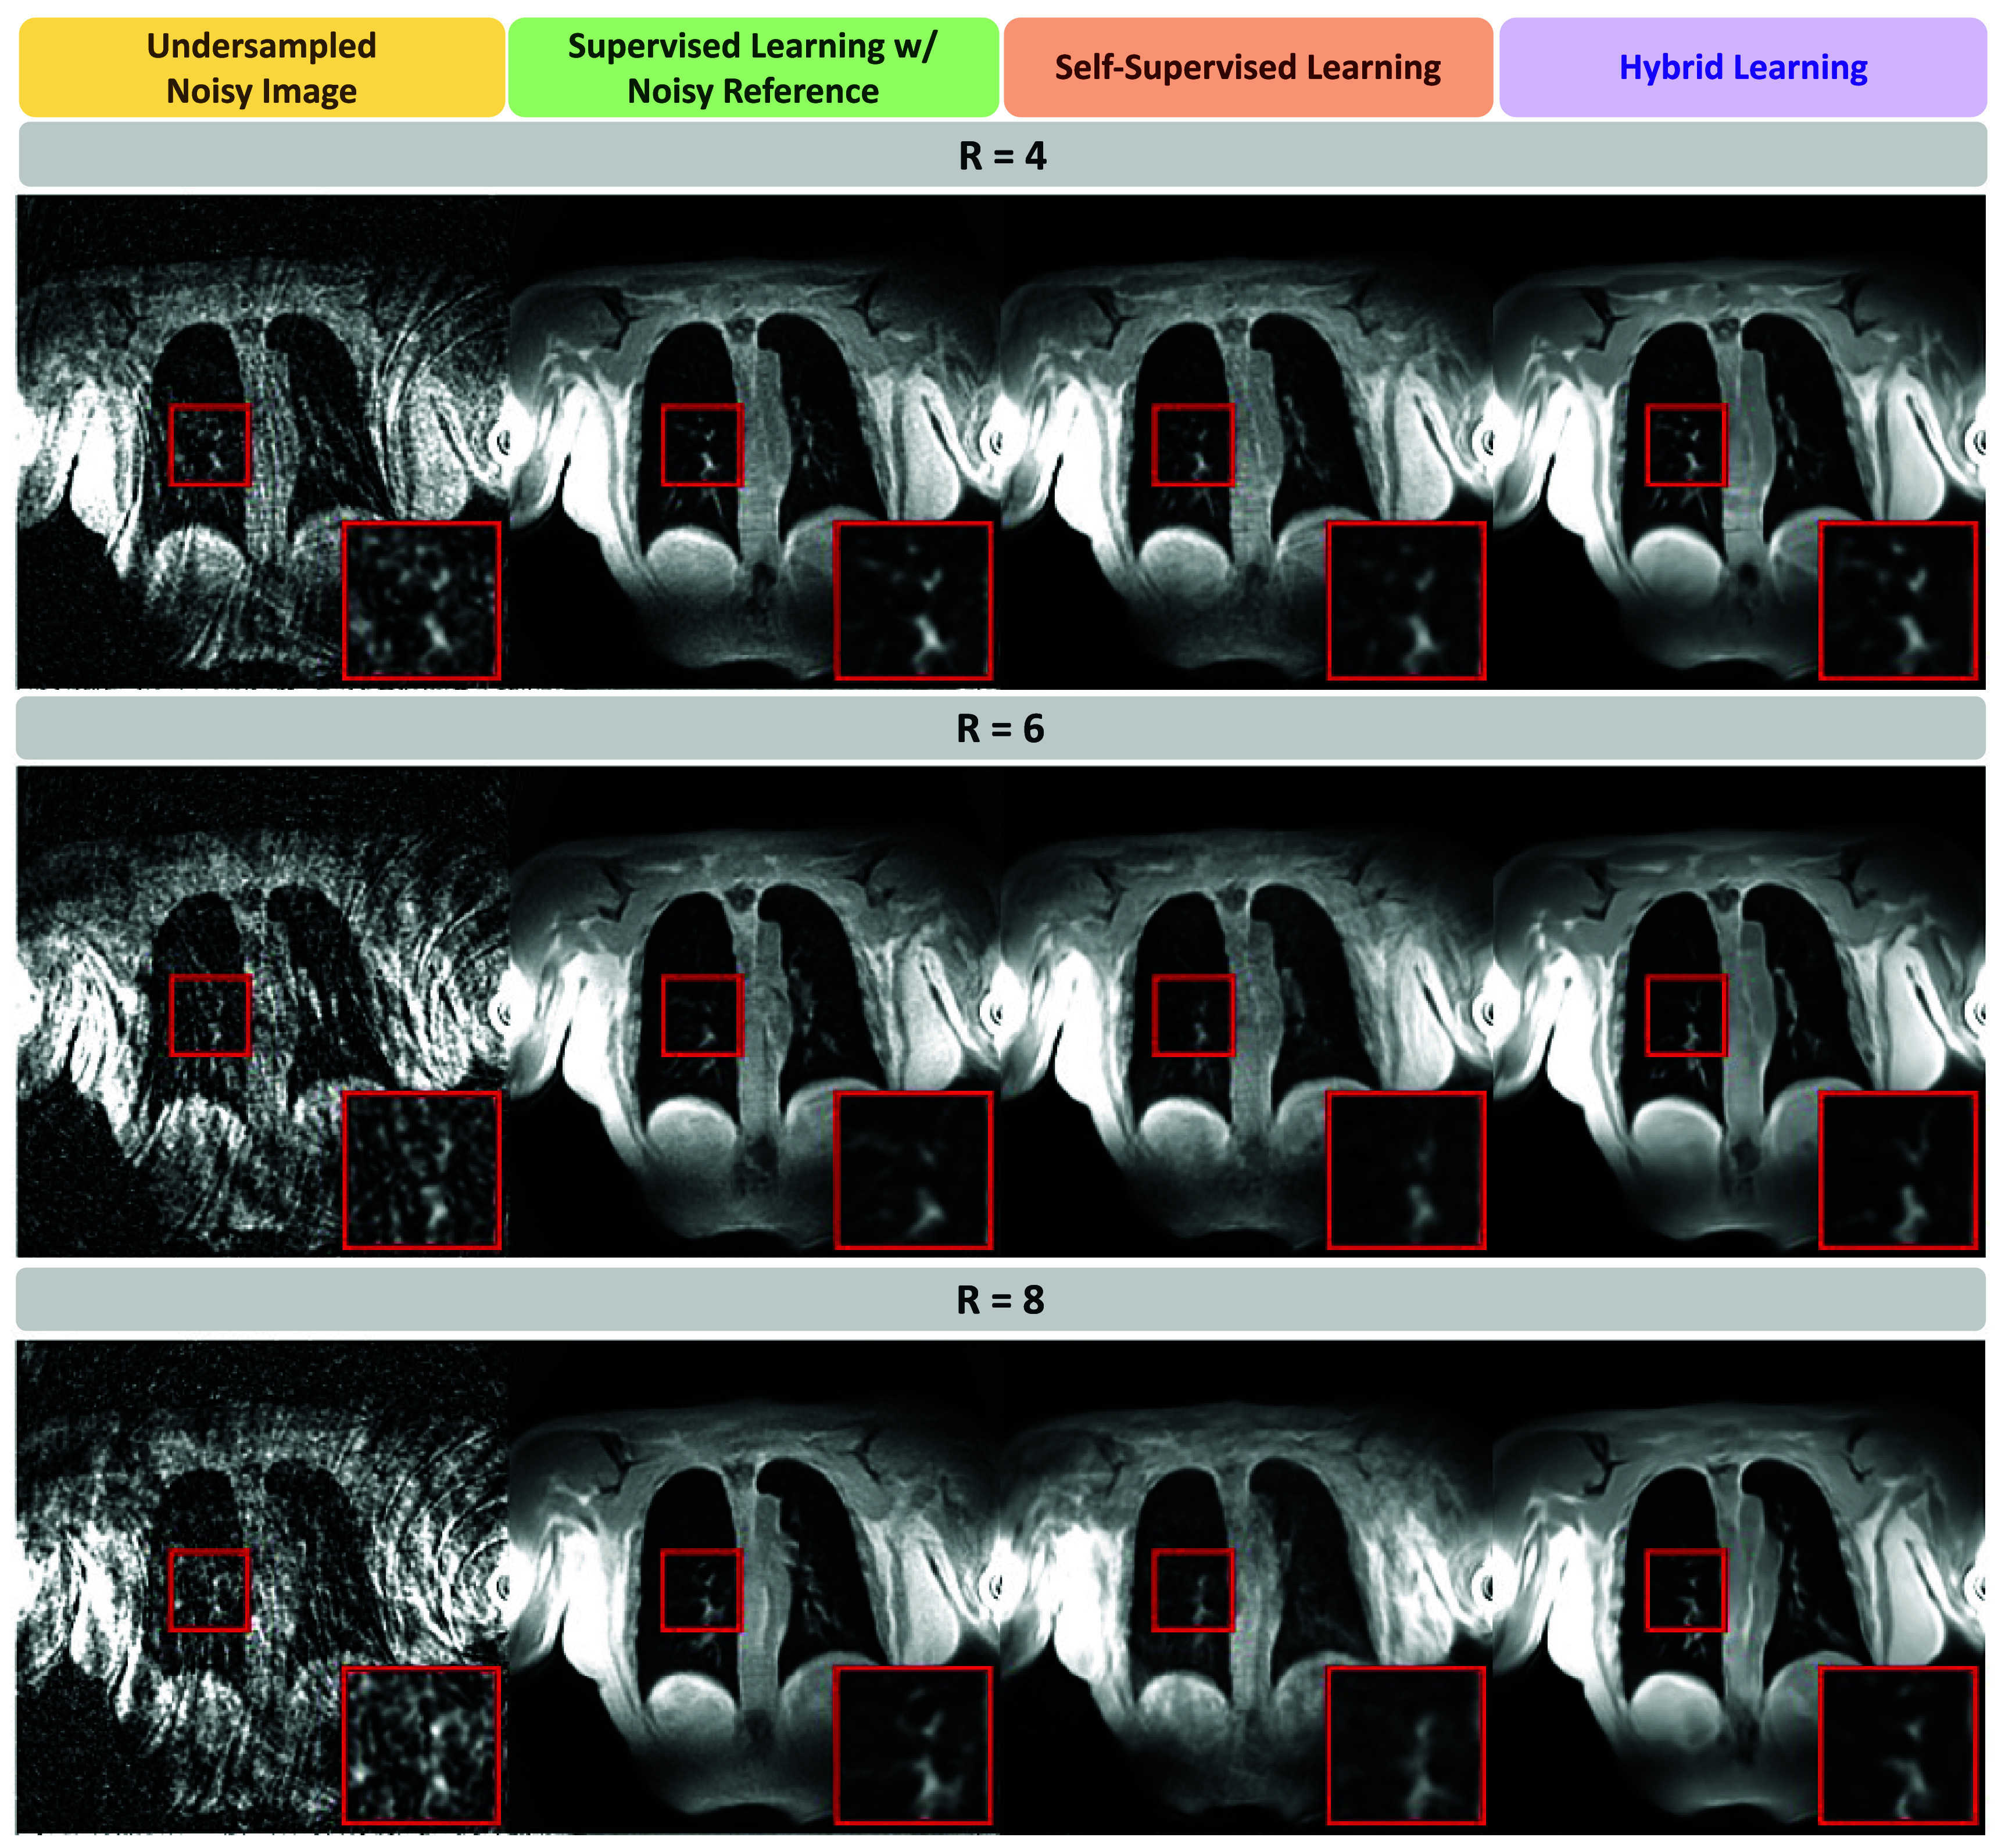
Lung MRI (0.55 T), spiral sampling at *R* = 4, 6, 8. The corresponding case from figure 8 at higher acceleration rates (*R* = 4, 6, and 8), comparing hybrid learning with supervised and self-supervised learning. Hybrid learning consistently provided improved image quality, with better preservation of structural details and fewer artifacts, even as reconstruction became more challenging for all methods at higher acceleration factors.
